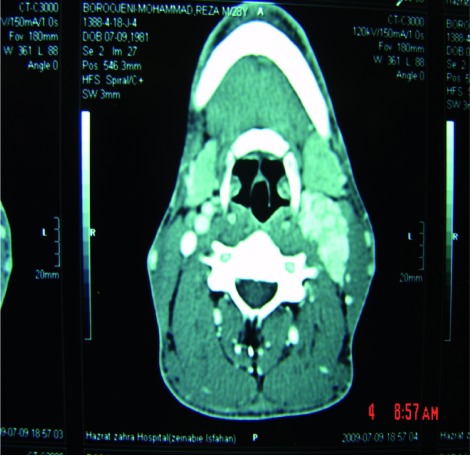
CT scan shows left neck mass.
